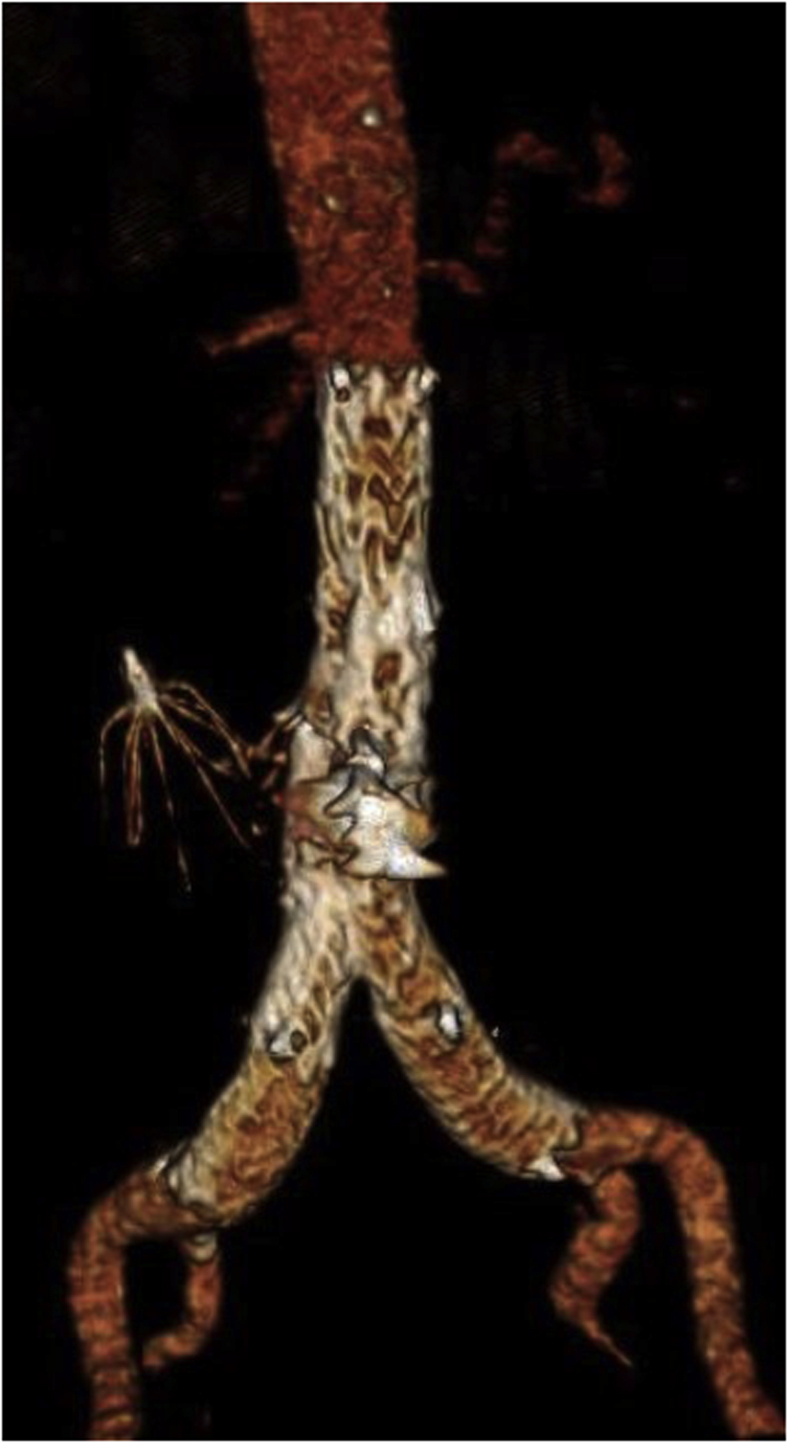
Postoperative computed tomography angiography (CTA) reconstruction of the aorta showing exclusion of the pseudoaneurysm with a stent graft.
